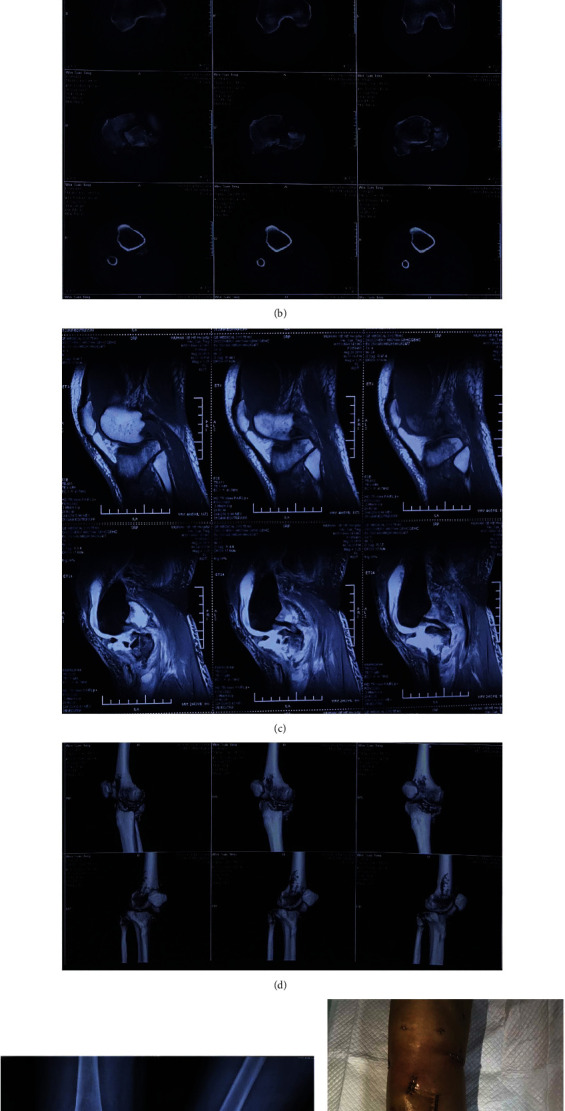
A 55-year-old male as a typical case of articular surface collapse. (a) X-rays of tibial plateau obtained before operation. (b) CT of tibial plateau obtained before operation. (c) MRI of the knee joint before operation. (d) CT of tibial plateau in 3D before operation. (e) X-rays of tibial plateau obtained right after operation. (f) Postoperative appearance of the affected limb.
